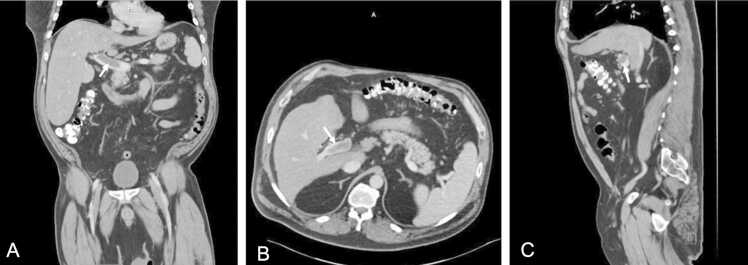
CT abdomen and pelvis with contrast at the emergency department a) coronal, b) transverse and c) sagittal: evidence of thrombus in the portal vein and superior mesenteric vein (white arrow).
